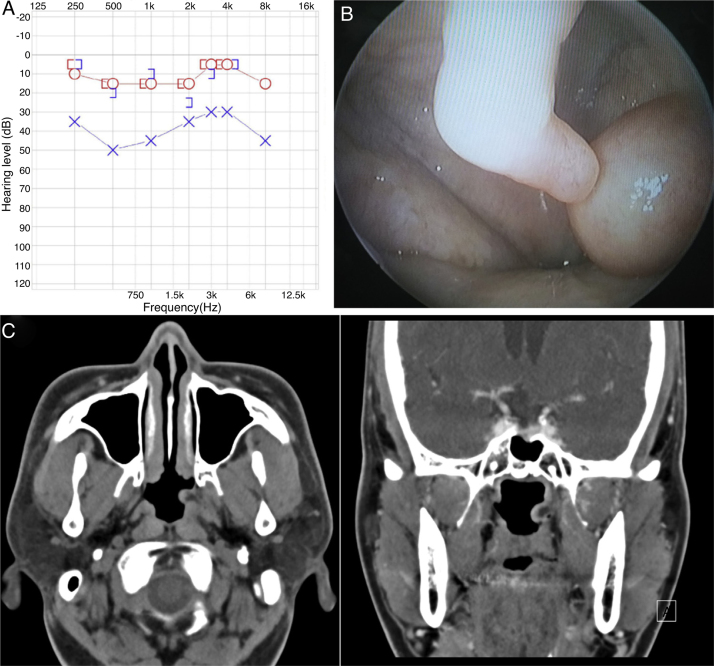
Preoperative findings. (A) Pure tone audiometry had 24 dB conductive hearing loss in the left ear. (B) Nasopharyngoscopic examination showed an about 10 × 5 smooth surfaced, round soft mass in the left side of nasopharynx, obstructing the opening of the left Eustachian tube totally. (C) Contrast-enhanced PNS CT shows a smooth-margined, mild enhancing, exophytic lesion in the opening of the left Eustachian tube. (left) Axial section, (right) coronal section.
